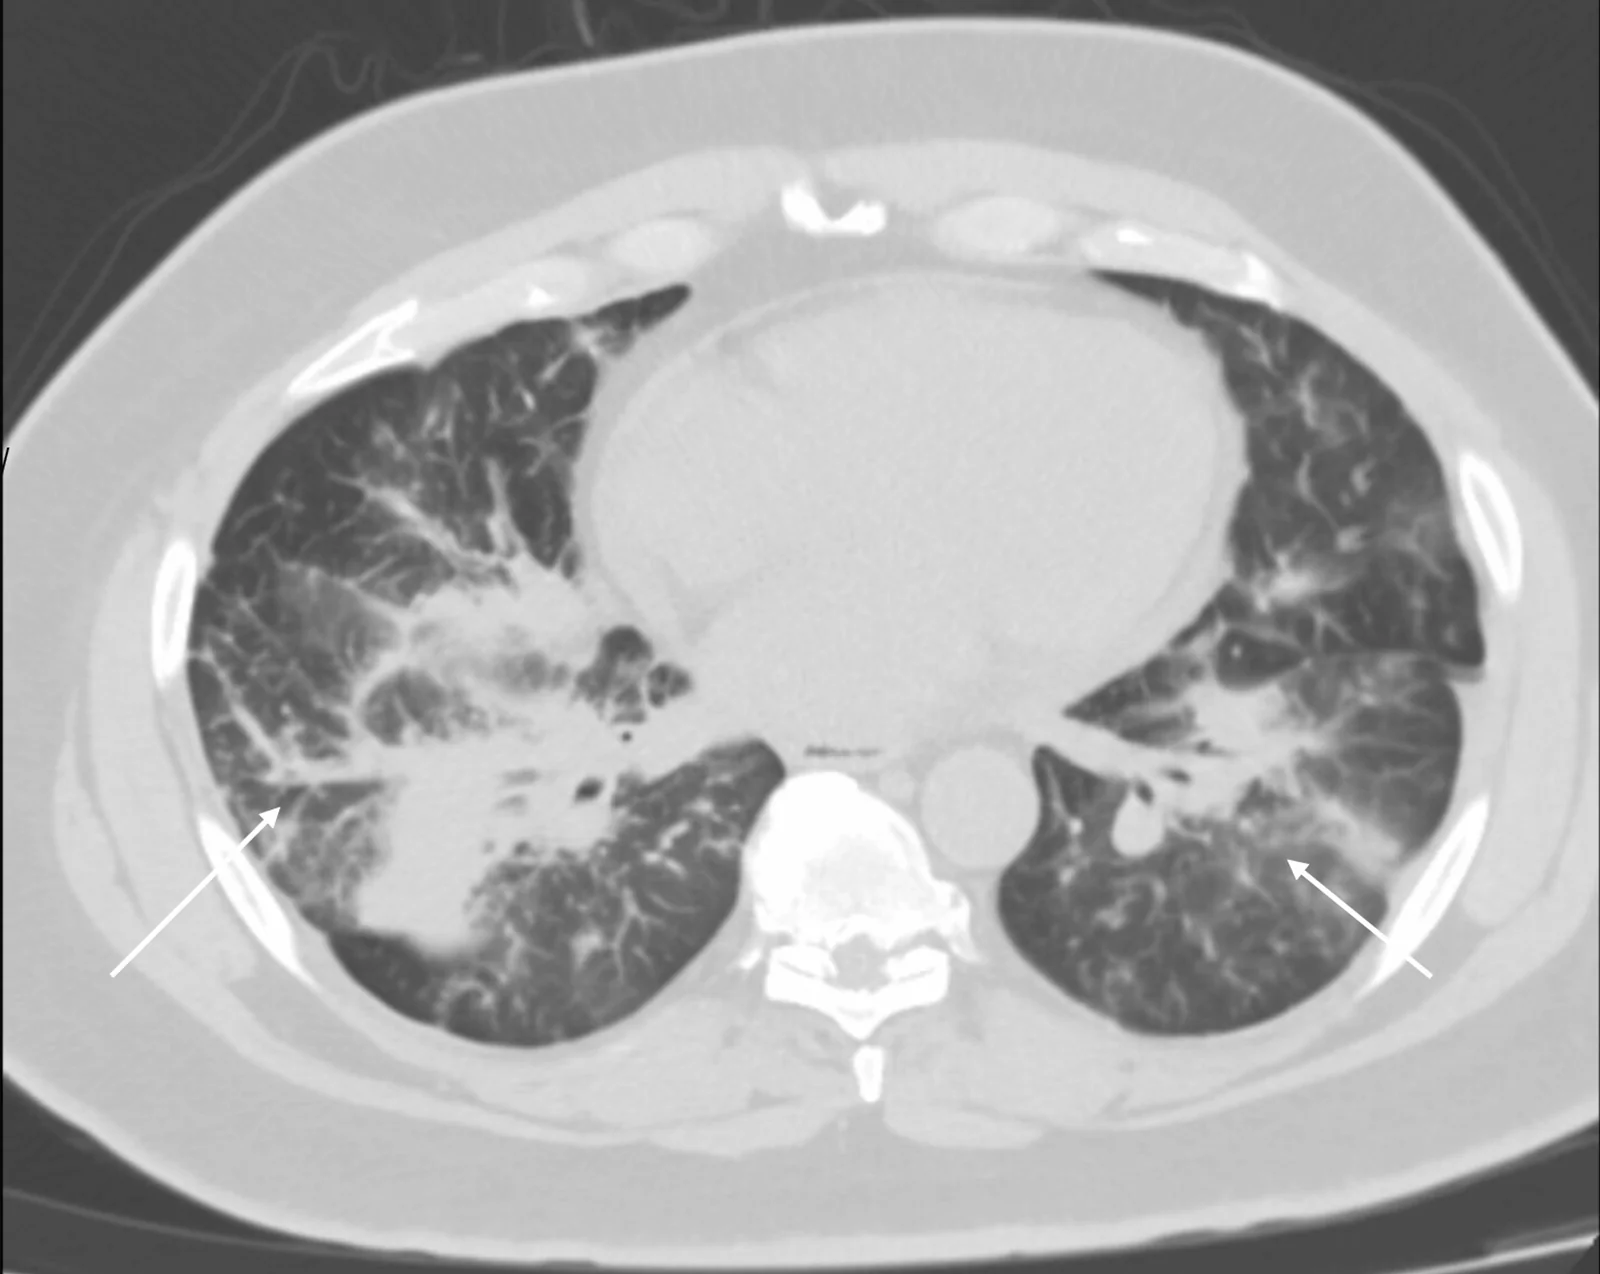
Chest computed tomography: Patchy and confluent pulmonary opacities in the lower lobes bilaterally (white arrows) with nodular interlobular septal thickening, extensive reticulation and groundglass opacities throughout both lungs.
